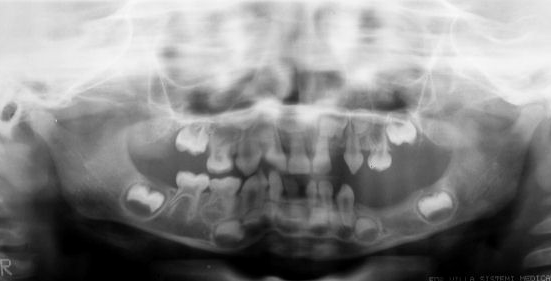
Orthopantomographic radiograph confirming the absence of deciduous (62, 74 and 75) and permanent teeth, mainly in the left mandibular area, and characterizing radicular and coronal changes.
